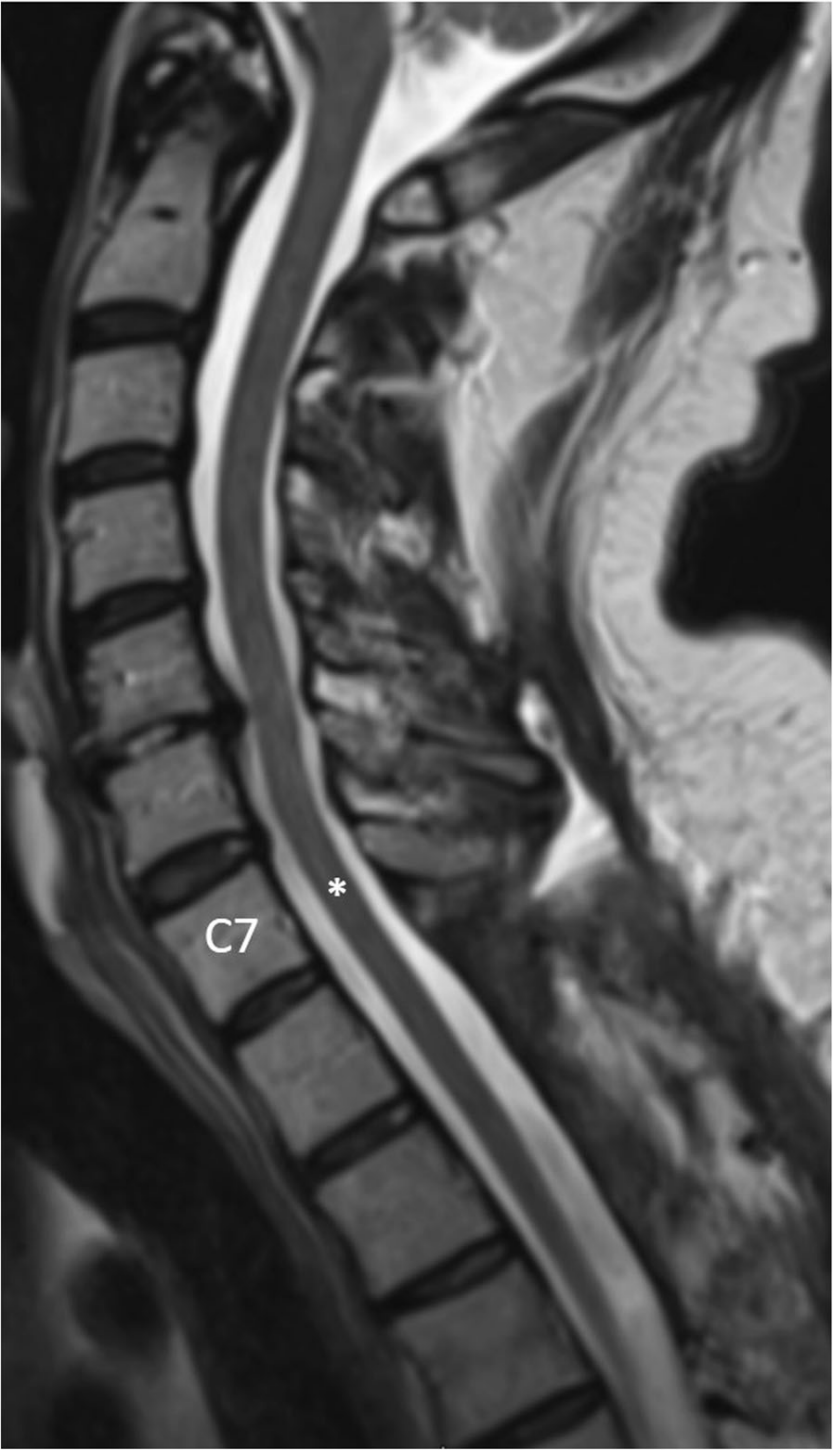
Post-operative sagittal T2-weighted MRI of the cervical spine demonstrates resolution of the epidural hematoma with decompression of the spinal cord (asterisk) at the C7 level. There has been restoration of normal spinal canal volume and there is no evidence of residual cord compression or myelomalacia. Note the absent visualization of the C7, T1, T2, and T3 spinous processes. The patient had partial C7, complete T1 and T2, and partial T3 laminectomy for decompression and hematoma evacuation
